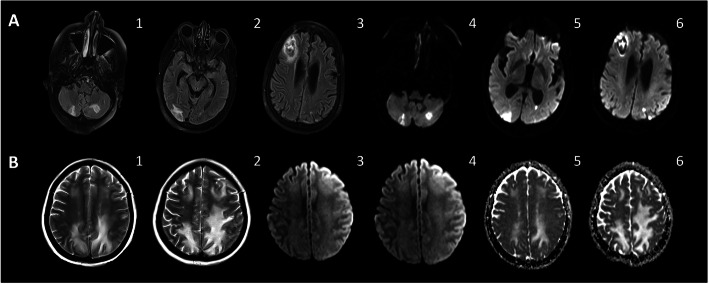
COVID-19 related stroke and posterior reversible encephalopathy syndrome. a Ischemic and hemorrhagic infarcts. A 73-year-old female presented with respiratory difficulty related to COVID-19 necessitating intubation. Following extubation and cessation of sedation, she remained persistently somnolent and was noted to have weakness of all extremities. Initial NIHSS was 12. Past medical history was notable for coronary artery disease, congestive heart failure, hypertension, and diabetes mellitus. Laboratory findings were notable for slightly elevated CRP (1.2 mg/dl) and significant elevation of ferritin (754 ng/ml) and D-Dimer (4184 ng/ml). Axial FlAIR MR images (1, 2, 3) demonstrate multifocal areas of signal abnormalities involving both cerebellar hemispheres, right occipital lobe, and right frontal lobe, with a hemorrhagic component (mostly involving right frontal region). Smaller foci of signal abnormality were present in the parietal cortices and centrum semiovale. Axial DWI (4, 5, 6) display multifocal areas of diffusion restriction consistent with acute ischemia. Hypercoagulopathy is the likely etiology of strokes. The patient had been on clopidogrel and low dose enoxaparin (DVT prophylaxis) before her stroke. Her neurological condition improved substantially with only minimal residual left hemiparesis and mild ataxia were noted at the first follow-up visit, which was several weeks after hospital discharge. b Posterior reversible encephalopathy syndrome (PRES). A 43-year-old SARS-CoV-2-positive female presented with respiratory distress that progressed rapidly to ARDS. Serological findings were notable for elevation of CRP (32 mg/dl), ferritin (259 ng/ml), and D-Dimer (7643 ng/ml). Post-extubation, she remained lethargic despite discontinuation of sedatives. She was slightly hypotensive to normotensive. The neurological exam showed lethargy, dysconjugate gaze, and triplegia. CSF analysis was entirely normal. Oligoclonal bands were absent. IgG index 0.8 (normal < 0.7). Notably, the neurological recovery was slow and concurrent with the return of serum inflammatory markers to normal levels. T2W MR images (1, 2) demonstrate bilateral parietal, and to a lesser extent frontal lobe, signal hyperintensities consistent with vasogenic edema. There is no corresponding high signal intensity on DWI (3, 4) or low signal intensity on ADC (5, 6) to suggest diffusion restriction. The findings are suggestive of PRES associated with high circulating markers of inflammation and coagulation pathways activation. The patient exhibited a slow, but steady, recovery concurrent with the reduction of serum levels of the inflammatory markers. This recovery was further enhanced by a 3-day course of intravenous pulse methylprednisolone (1000 mg/day). She was discharged home with only mild cognitive and motor impairment
